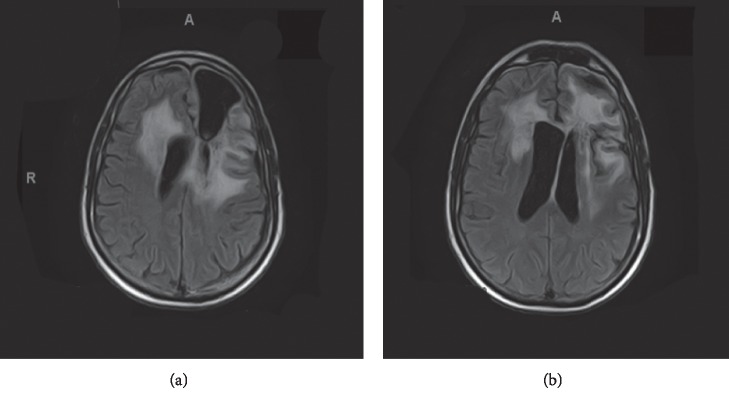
Posttherapy T2 FLAIR, May 2018. (a) and (b) showing improvements in cerebral edema seen in comparison to March 2018 imaging in Figure 2.
